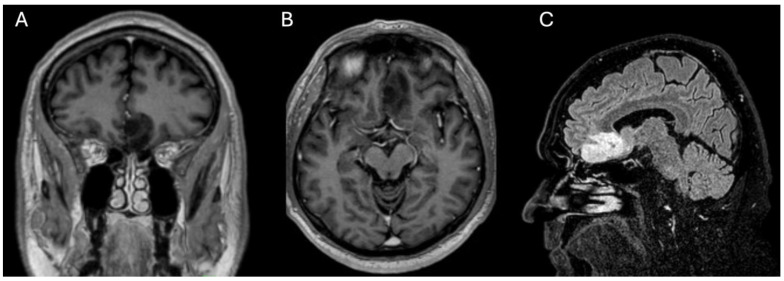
Head magnetic resonance (MRI) imaging of a patient with a low-grade glioma of left orbitofrontal cortex. (A)—coronal T1 contrast-enhanced MRI; (B)—axial T1 contrast-enhanced MRI; (C)—sagittal 3D brain volume imaging with enhanced water (VIEW) fluid-attenuated inversion recovery (FLAIR).
